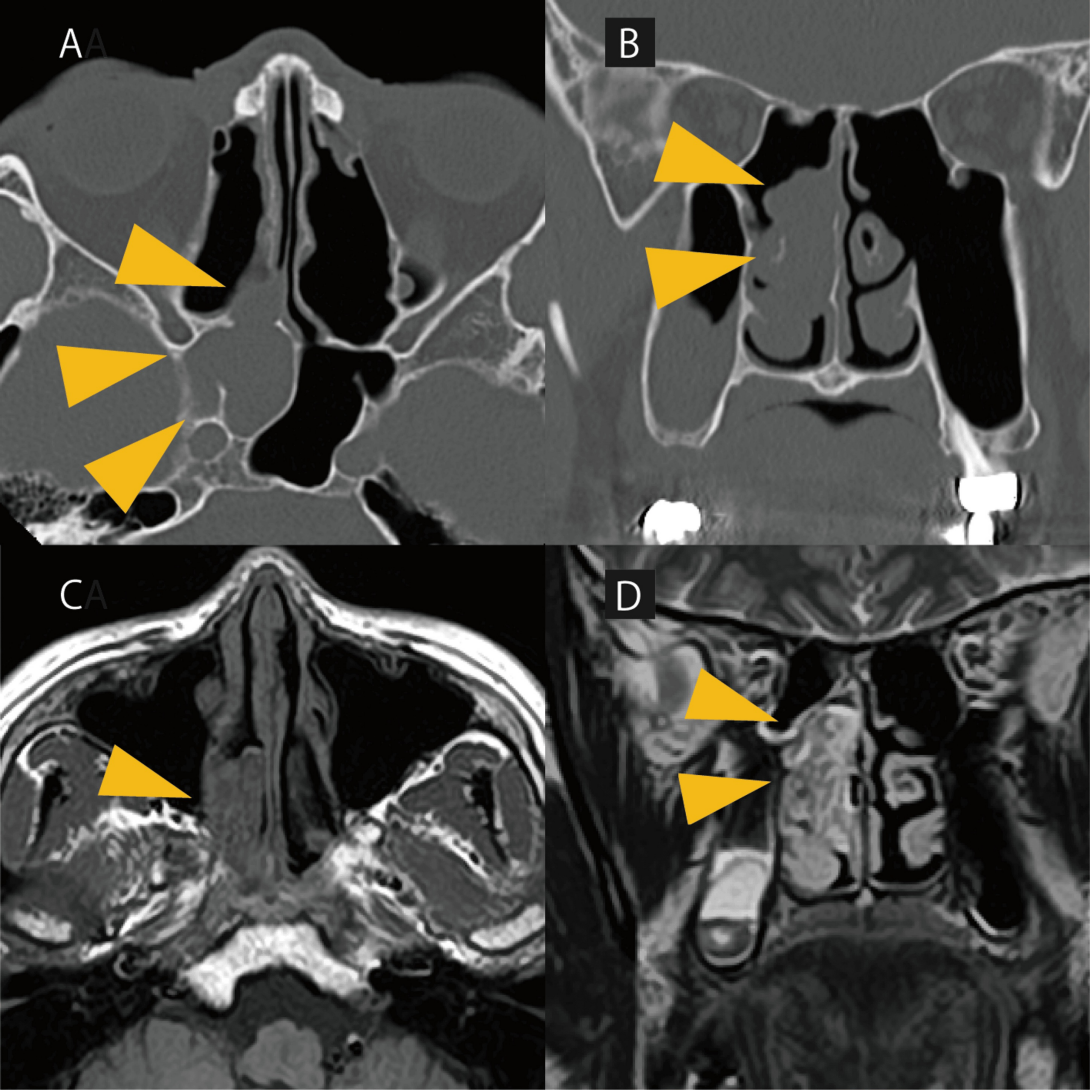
Initial radiological findings (A, B) Axial computed tomography (CT) of the paranasal sinuses demonstrating soft tissue opacification in the sphenoid sinus and its anterior extension. No evident osseous destruction is observed. (C, D) Magnetic resonance imaging (MRI) of the sinuses revealing an isointense mass on T1-weighted images and a heterogeneous iso- to hyperintense mass on T2-weighted images, exhibiting a characteristic cerebriform pattern. Yellow triangles indicate the tumor on both imaging modalities.
